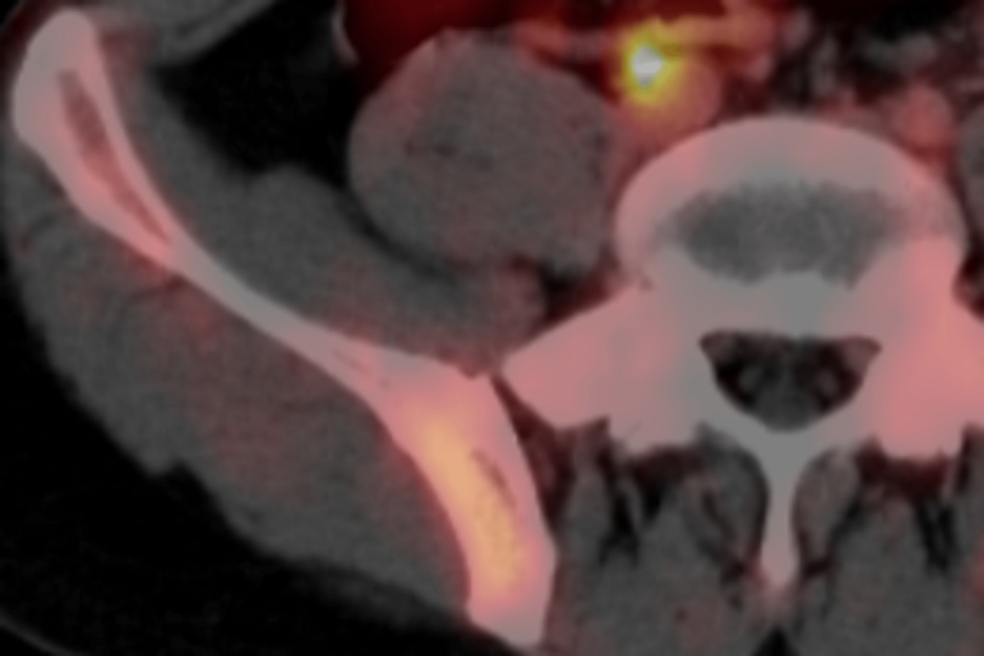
PET-CT after the chemotherapy
